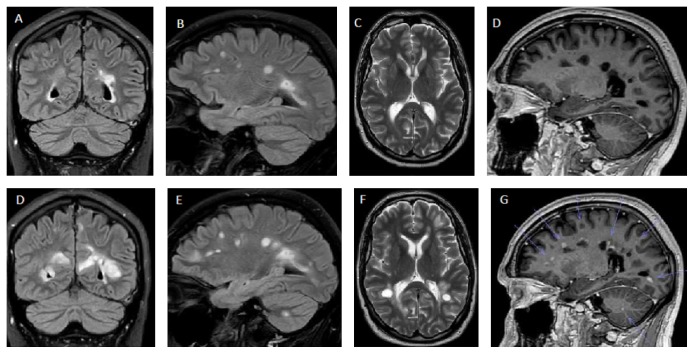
MRI before cessation of fingolimod in September 2016 (upper row) and 19 days after infusion of rituximab (July 2017; lower row). Coronal (A/D ) and sagital (B/E) flair and coronal T2 (C/F) images show several new and enlarging lesions after switch to rituximab. Arrows indicate contrast enhancement on sagital T1 images (D/G).
